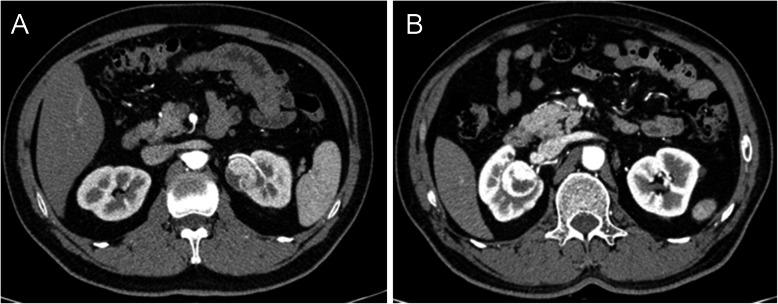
a Renal-enhanced computed tomography scan showing posterior hilar tumor (left side). b Renal-enhanced computed tomography scan showing posterior hilar tumor (right side)
